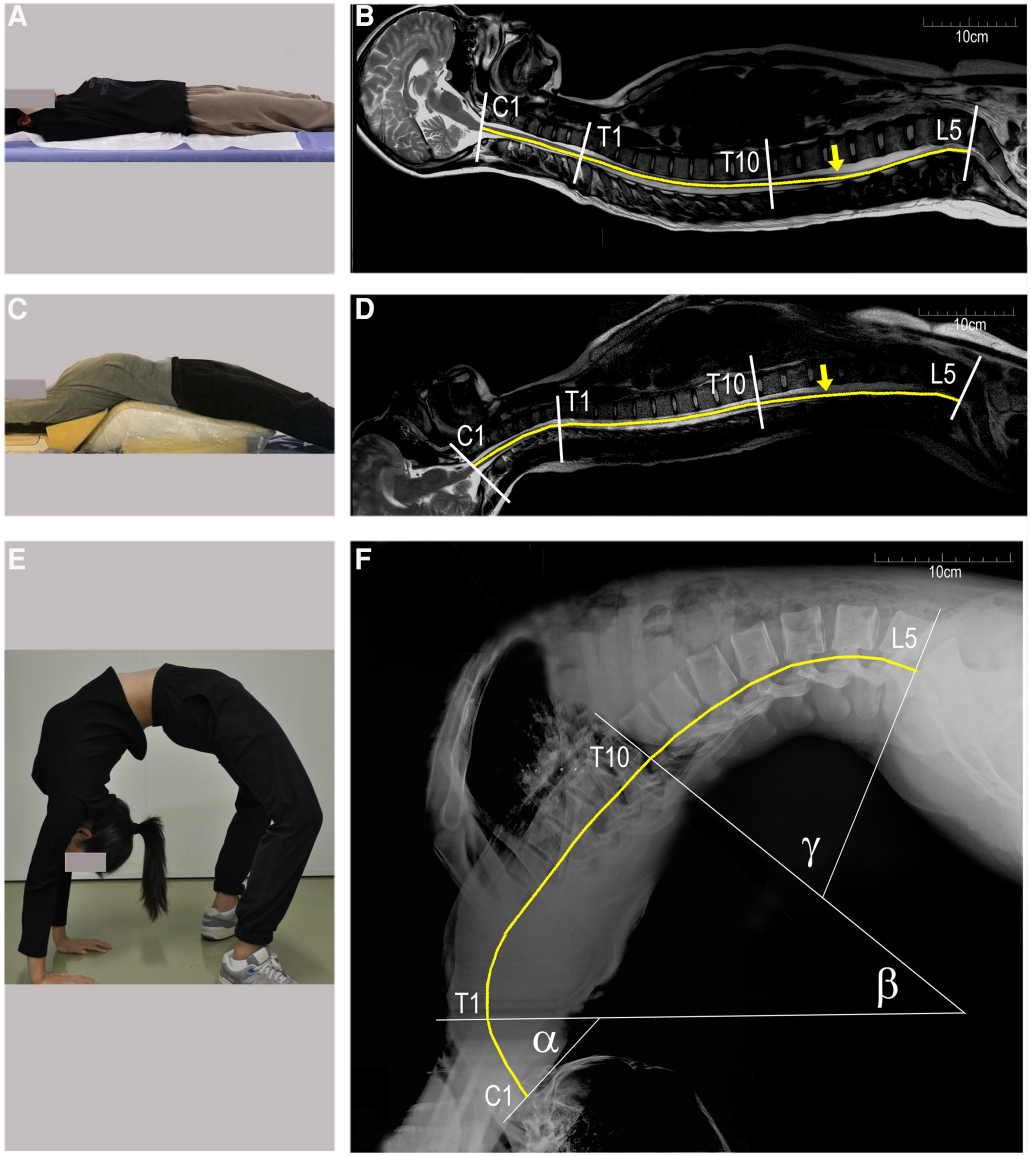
Changes in the spinal length of a healthy female dancer (16 years of age, 168 cm in height). (A-D) sagittal MRI of the whole spine in the supine and partial backbend positions; (E and F) lateral x-rays of a full backbend. The spine is divided into three segments: C1–C7 segments with angle α, T1–T10 with angle β, and T11–L5 with angle γ (F) the end of the conus medullaris is marked by yellow arrows in (B and D). The length of the spinal cord is estimated by the curved yellow lines. C1, first cervical vertebra; T1, first thoracic vertebra; L5, fifth lumbar vertebra.
